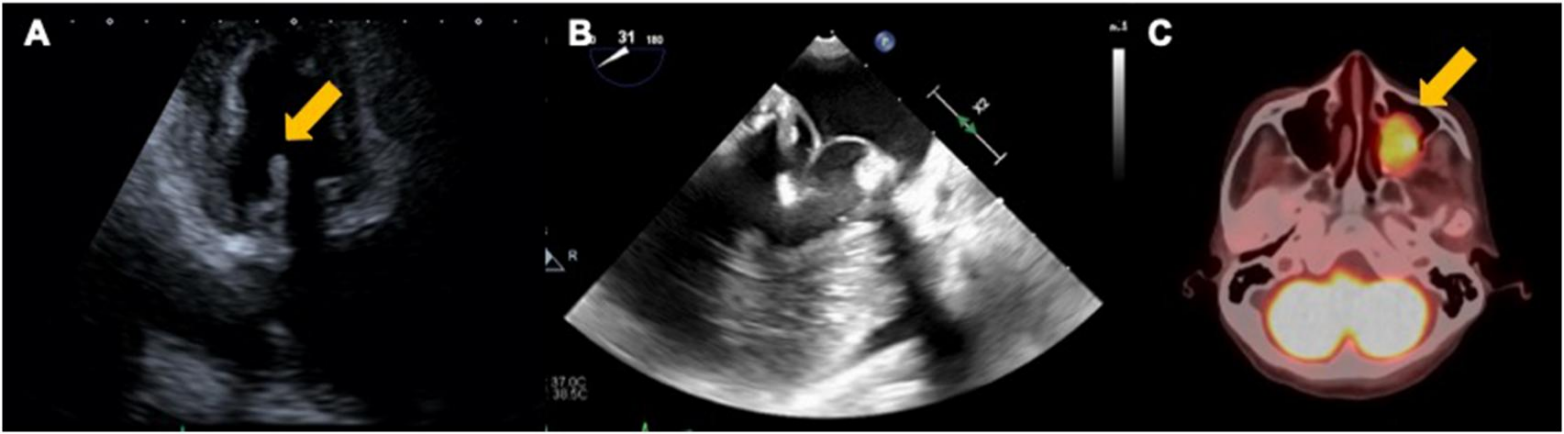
Transthoracic Echocardiography Showing Recurrent NBTE with the Mobile Vegetation Attached to Bioprosthesis (A). Transoesophageal Echocardiography Showing the Disappearance of Vegetation after Anticoagulant Therapy (B). Positron Emission Tomography-Computed Tomography Showing Increased Uptake of Fluorodeoxyglucose in the Right Maxillary Sinus (C)
